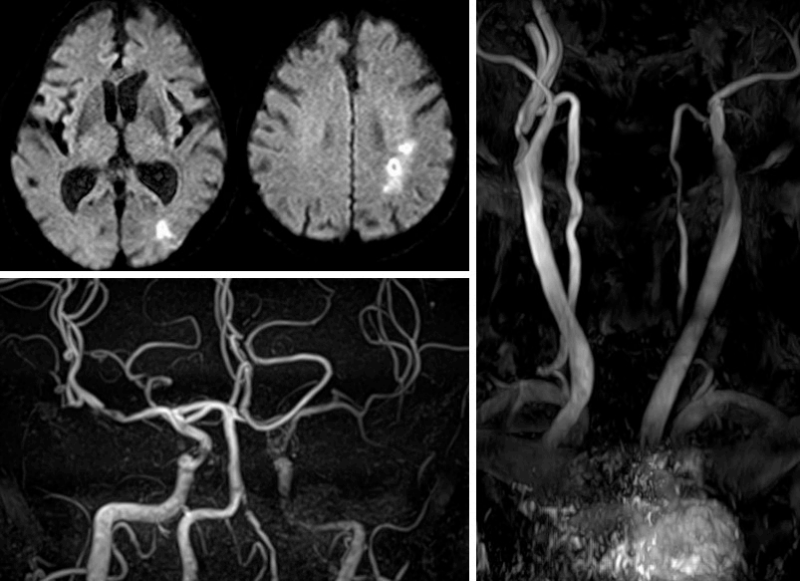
Magnetic resonance imaging of the head showing a cerebral infarct in the left hemisphere and magnetic resonance angiography showing severe stenosis of the left internal carotid artery.
